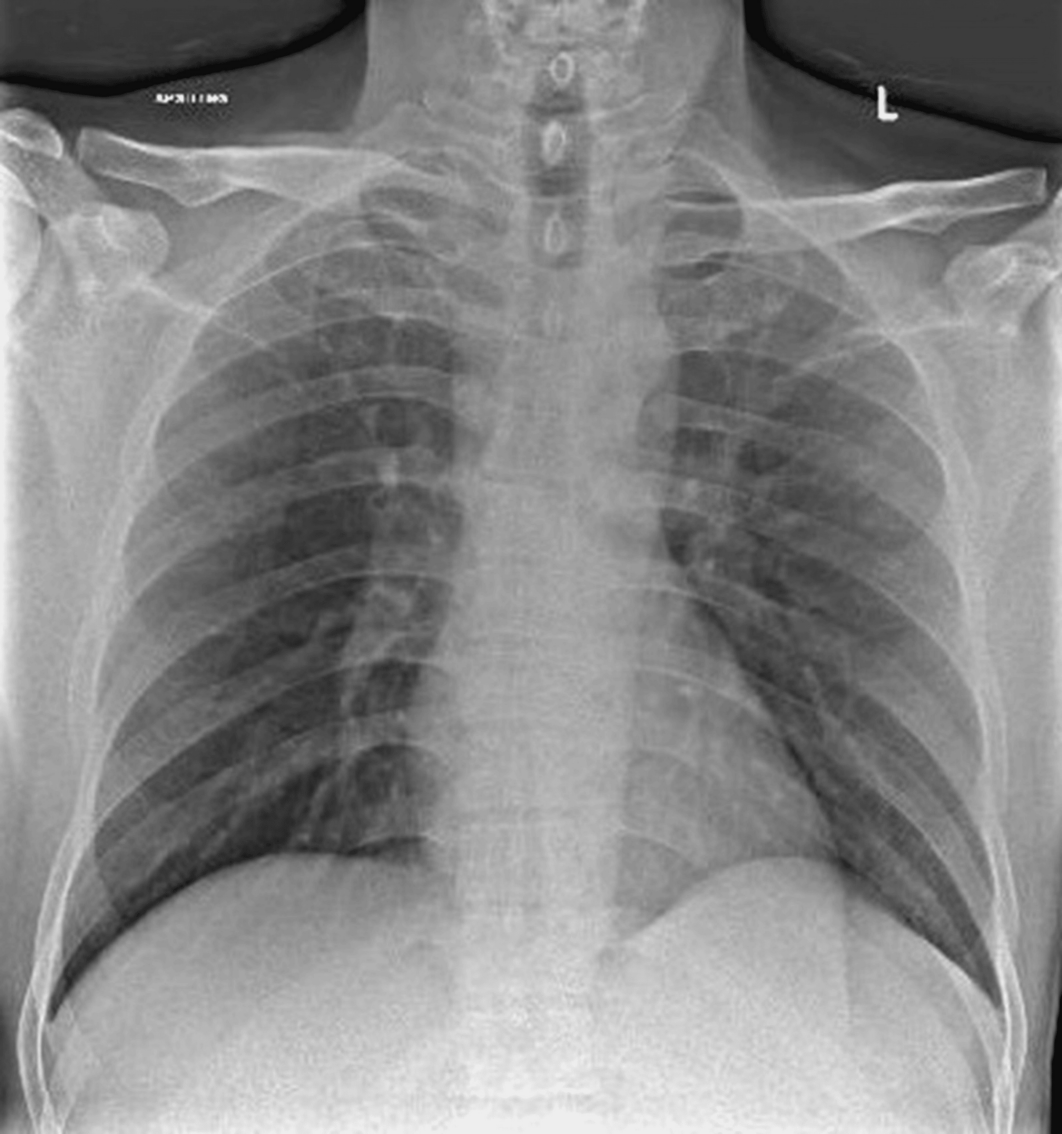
Chest radiograph obtained one week after CT scan of the thorax was performed
